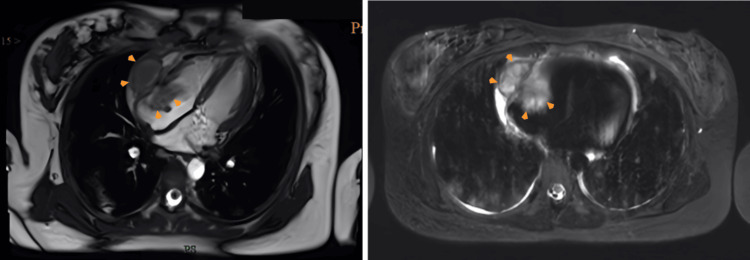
Cardiac MRI. (Left panel) Four-chamber cine imaging. Hyperintensity was demonstrated in the mass region with FS T2-weighted, combined with pericardial effusion. (Right panel) A mass (5 × 3 × 4 cm) (orange arrowheads) is located in the lateral wall of the right atrium (RA) and pericardial space. A portion protruded into the RA cavity. Mass borders are irregular. FS, fat saturation; MRI, magnetic resonance imaging
